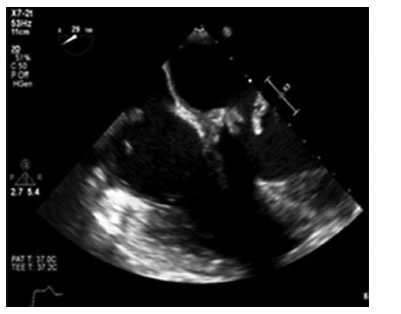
Transesophageal echocardiogram with vegetation on and dehiscence of the bioprosthetic mitral valve.
